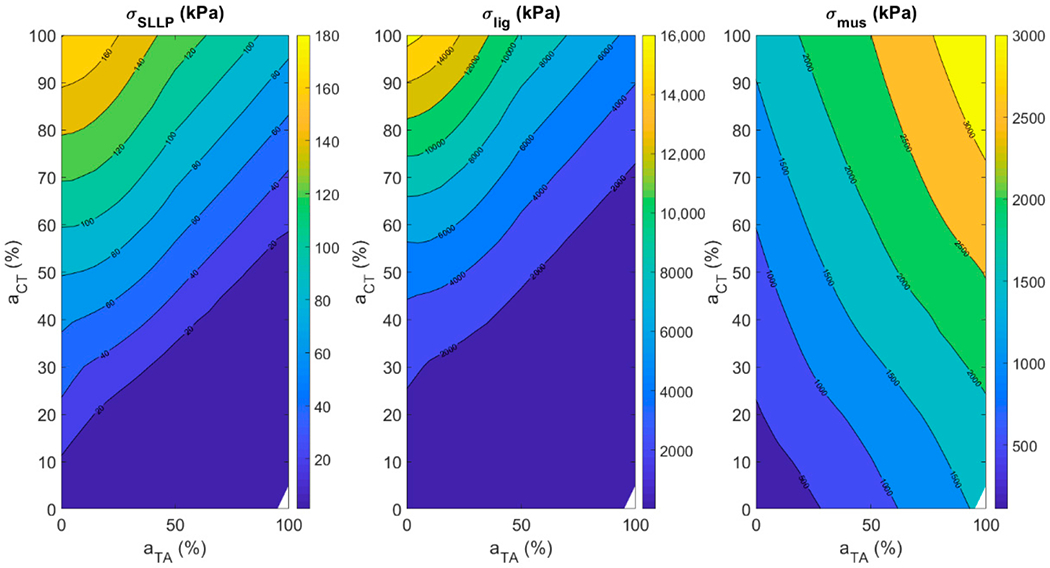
Muscle activation plots for fiber stress in (left) SLLP layer, (center) ligament layer, and (right) TA muscle with respect to TA–CT muscle activations.
